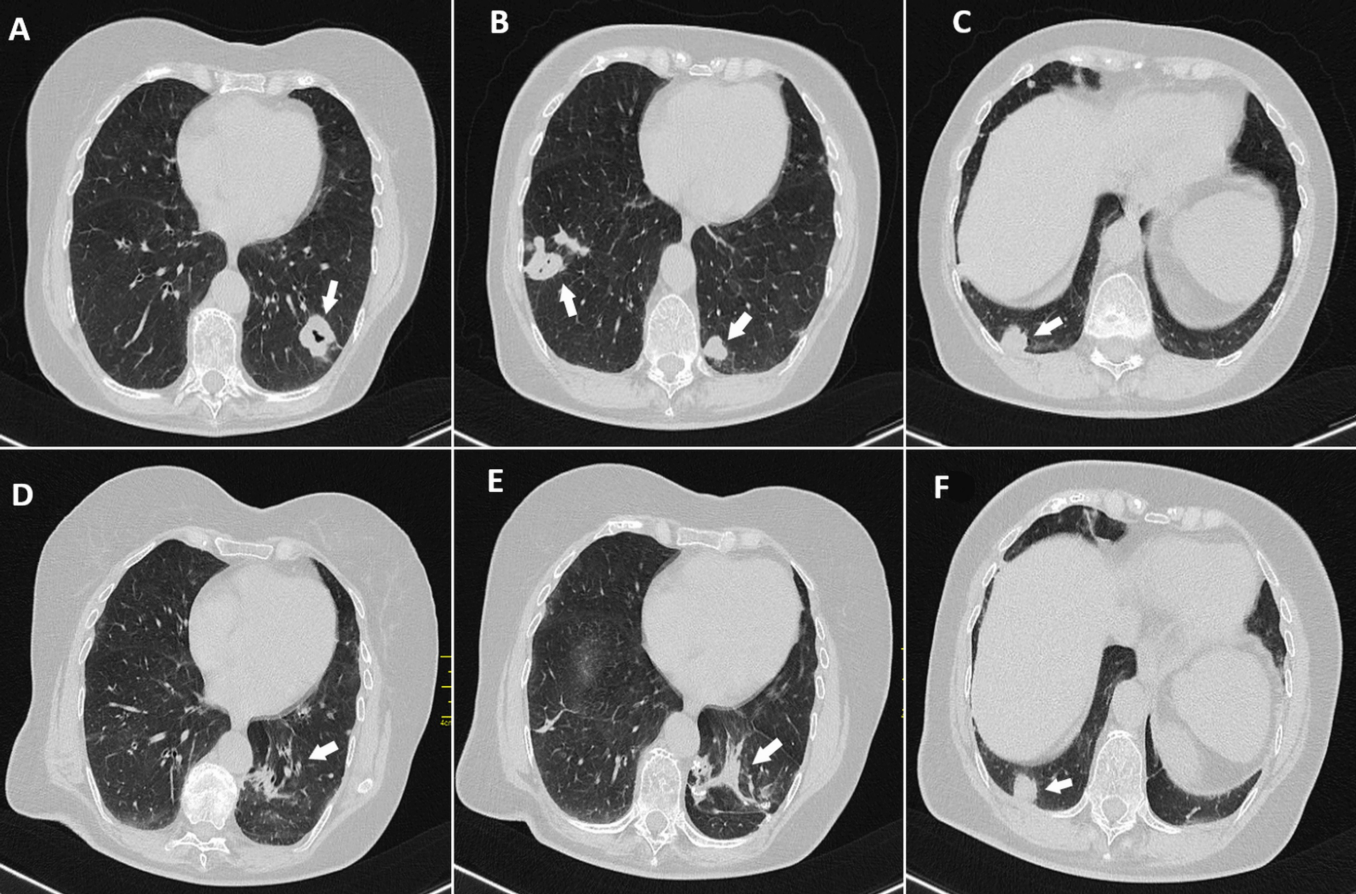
Contrast-enhanced chest CT scan (axial lung window) after hospital admission (A-C), and follow-up CT thorax performed eight months after the previous examination (D-F) (A) Cavitary nodule in the left lower lobe measured 36 × 26 mm. (B) Cavitary nodule in the right lower lobe measured 28 × 20 mm, as well as a solid nodule in the left lower lobe. (C) Solid nodule in the right lower lobe. (D-E) Left basal fibrotic changes containing calcifications. (F) A remaining solid nodule in the right lower lobe.
